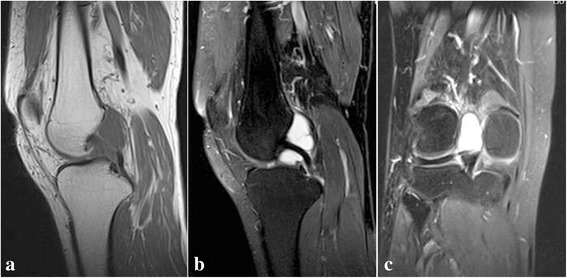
MRI images of the PCL cyst. a Sagittal T1W MRI: encasing the PCL with homogeneous low-signal intensity. b Sagittal T2W MRI: encasing the PCL with high-signal intensity. c Coronal T2W MRI: high-signal intensity
